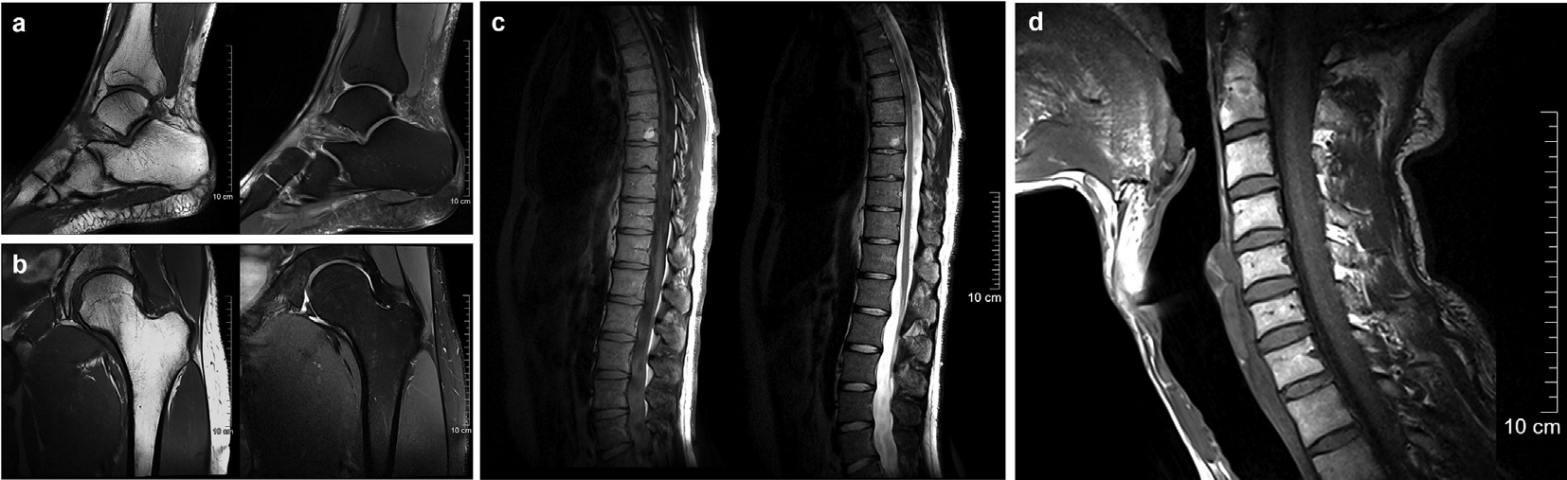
In vivo MR images acquired with an 8- or 16-channel ModFlex coil of the a) right ankle: T1-weighted (left) and PD-weighted (right) image, b) left hip: T1-weighted (left) and PD-weighted (right) image, c) spine: T1-weighted (left) and T2-weighted (right) image, d) neck: T1-weighted image. MR sequence details and parameters are given in Table I.
